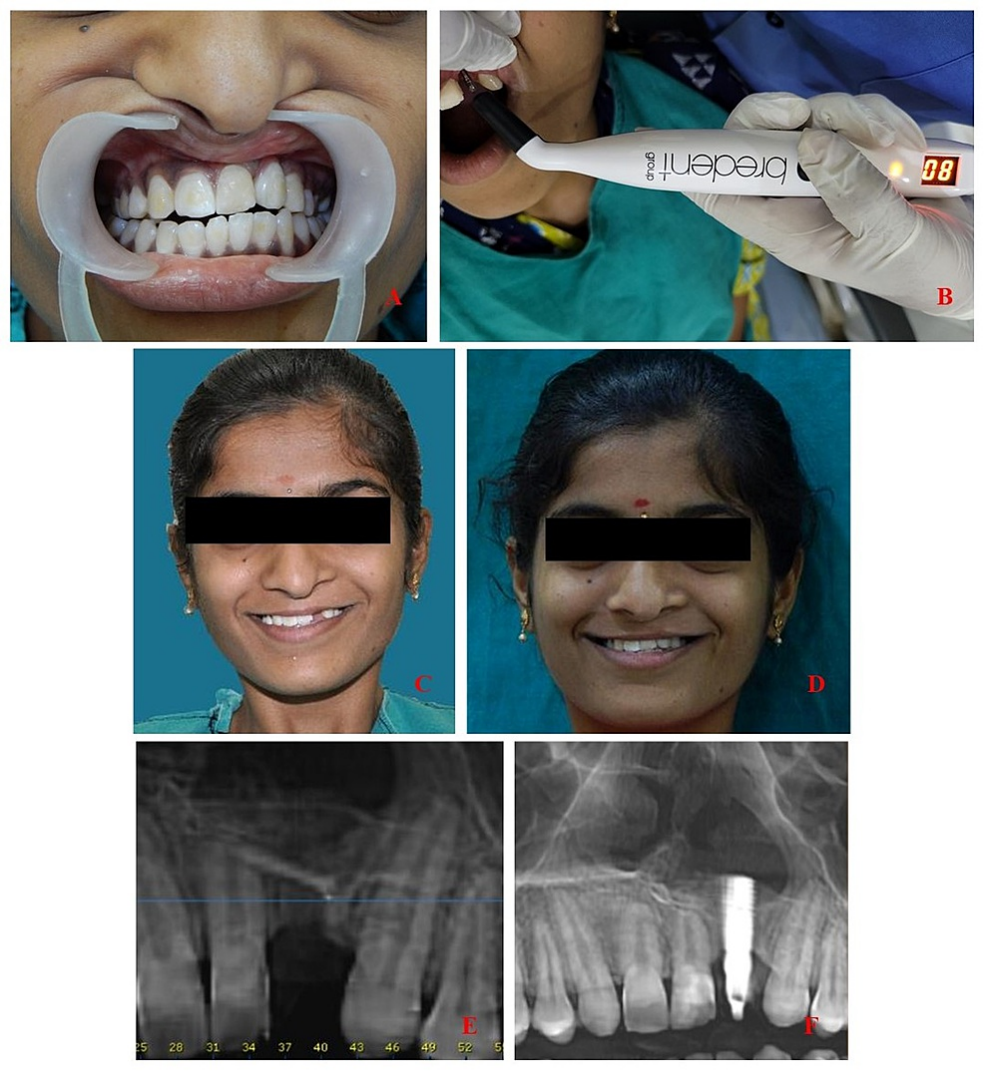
A: Definitive prosthesis in place. B: ISQ assessment prior to definitive crown cementation. C and D: Improvement in patient’s esthetics following dental implant-based rehabilitation. E and F: Pre- and post-operative CBCT images. ISQ, implant stability quotient; CBCT, cone-beam computed tomography.
